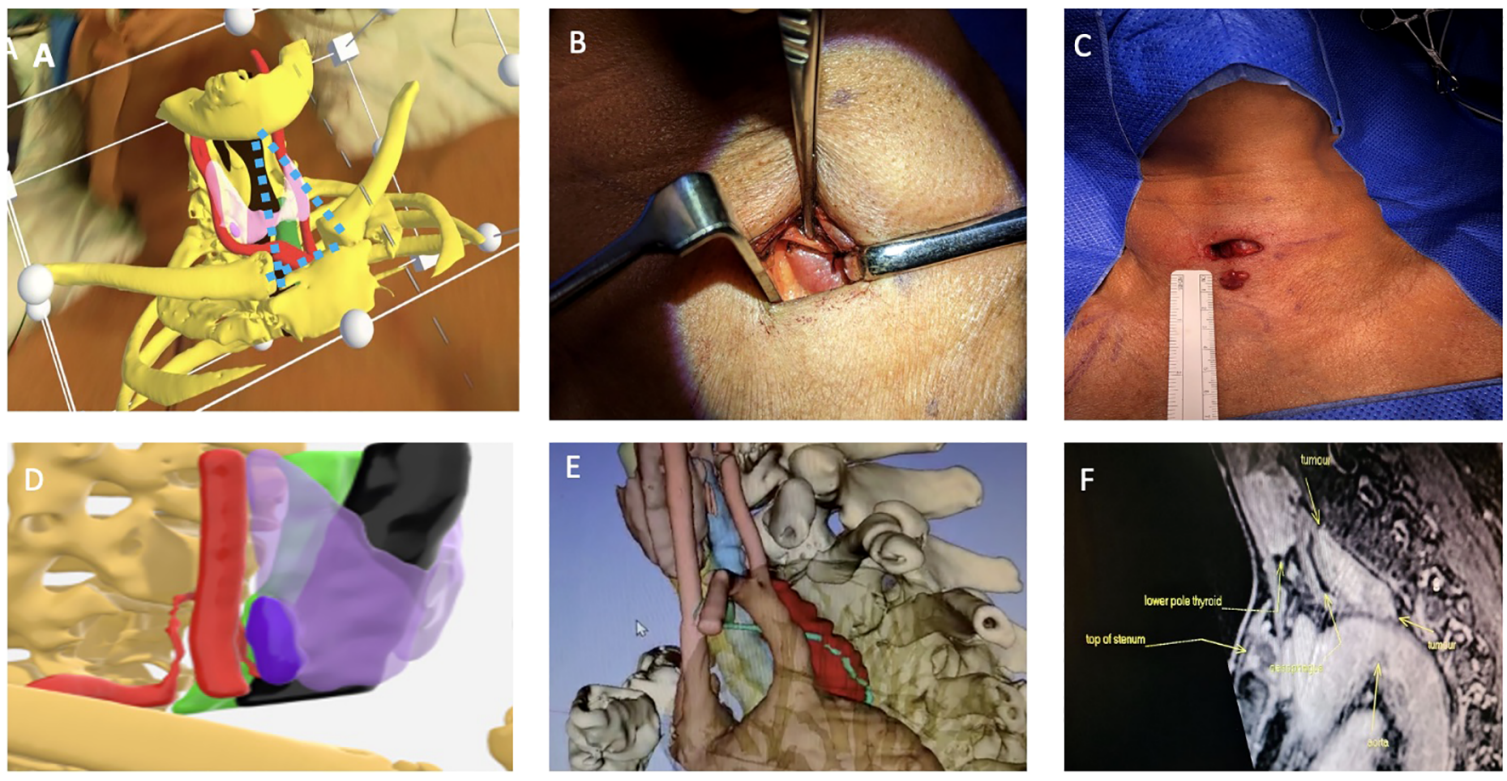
Top row (A) Surgeon’s view from the right side of the operating table of 3D model superimposition on patient’s neck using Microsoft HoloLens2,the parathyroid adenoma (Right lower pink structure), thyroid (light purple in transparency), carotid artery (red) and bones (yellow), imaginary triangulation dotted lines in blue. (B) Shows incision placement and immediate location of the parathyroid adenoma with minimal dissection and no subplatysmal flap elevation. (C) Postoperative excision with maintained wound length as there was no need for excessive retraction. Bottom row (D) It shows side-view of the 3D model where ITA and its branch which supplies the parathyroid adenoma are reconstructed, in this model, thyroid gland (light purple), right parathyroid adenoma (deep purple), trachea (black) and carotid artery (red). (E) shows a very large parathyroid tumour in the prevertebral space closely related to the aortic arch and the thoracic duct (lime green). (F) MRI sagittal view shows relation of parathyroid adenoma to aortic arch but thoracic duct was more difficult to visualise. The thoracic duct was identified and ligated at the start of the dissection to avoid a thoracic duct injury and a chyle leak.
